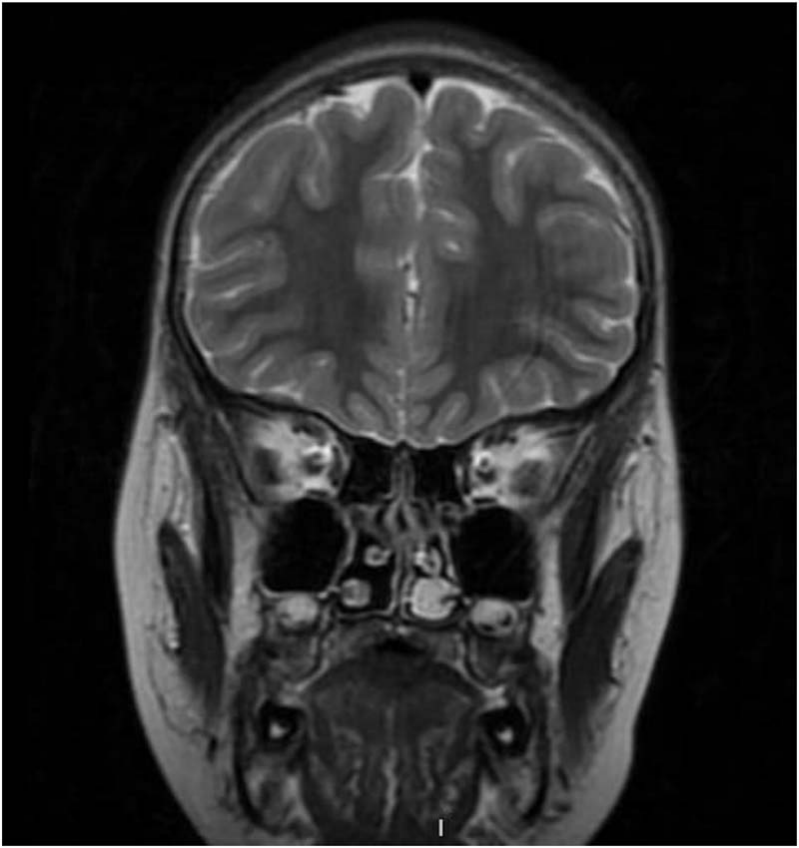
Pre-operative coronal T2-weighted brain MRI showing high signal intensity left-sided intra-nasal lesion.
